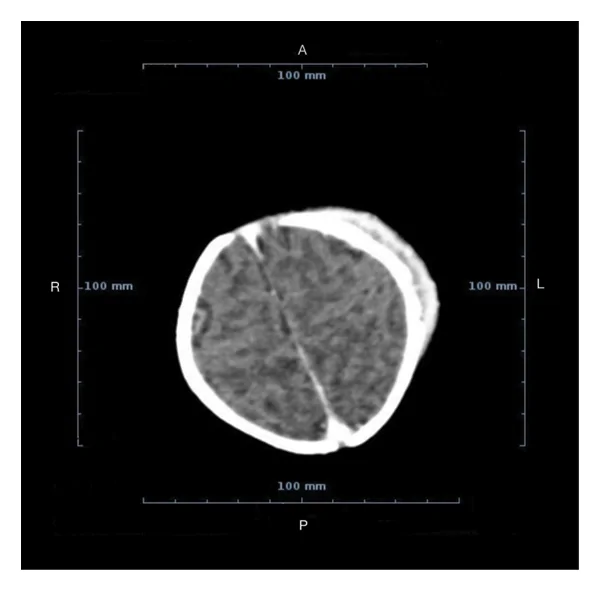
CT head with contrast (brain window); the yellow arrow shows the unaffected brain parenchyma, and the red arrow shows the irregular thickness of the infected bone underlying the infected subgaleal hematoma.
